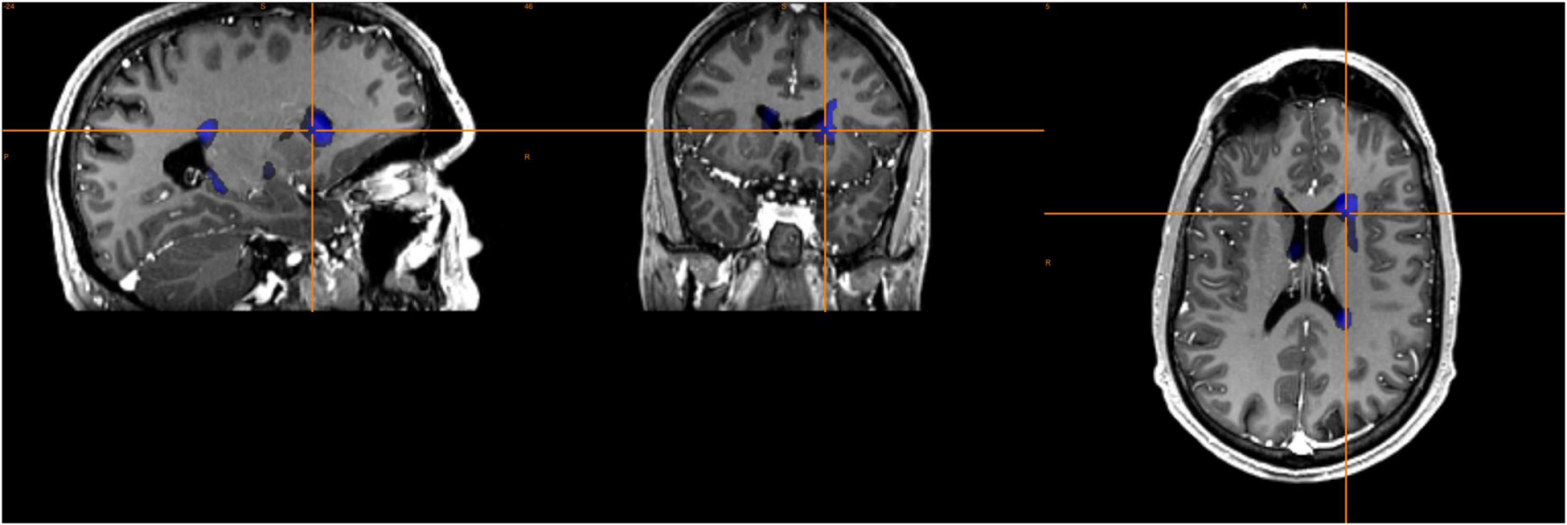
Computer-aided subtraction SPECT imaging in a patient with severe dystonia. The patient was 35 years old with long history of cervical dystonia and choreoathetotic movements. We obtained Technetium 99 m Neurolite SPECT scan of the brain in this patient with awake state and under anesthesia, respectively. Subtraction SPECT imaging had shown a hypermetabolic left caudate (blue), which is helpful for DBS target selection.
